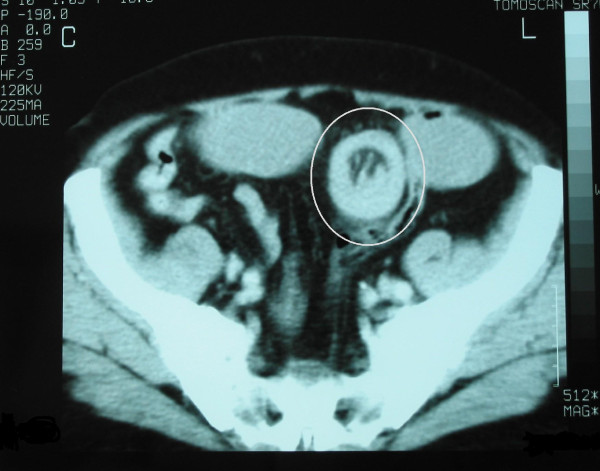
Abdominal CT scan. A loop of small intestine (that very probably belongs in the proximal part of ileum) in the left lesser pelvis, with abnormal wall thickening (approximately 10 mm), and a signet-ring-like unilateral thickening (marked by circle).
